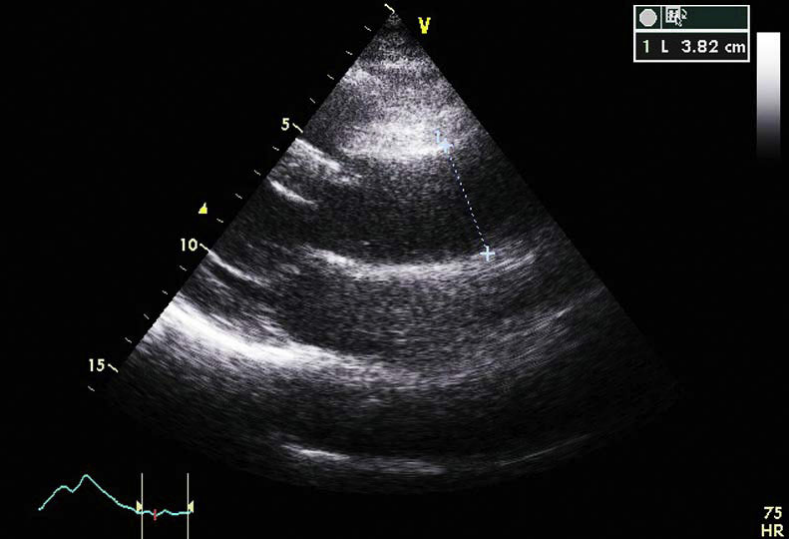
Echocardiography image-aneurysm. A left parasternal view of the ascending aorta in echocardiography examination of an affected family member with an aneurysm radius of 3.8.
